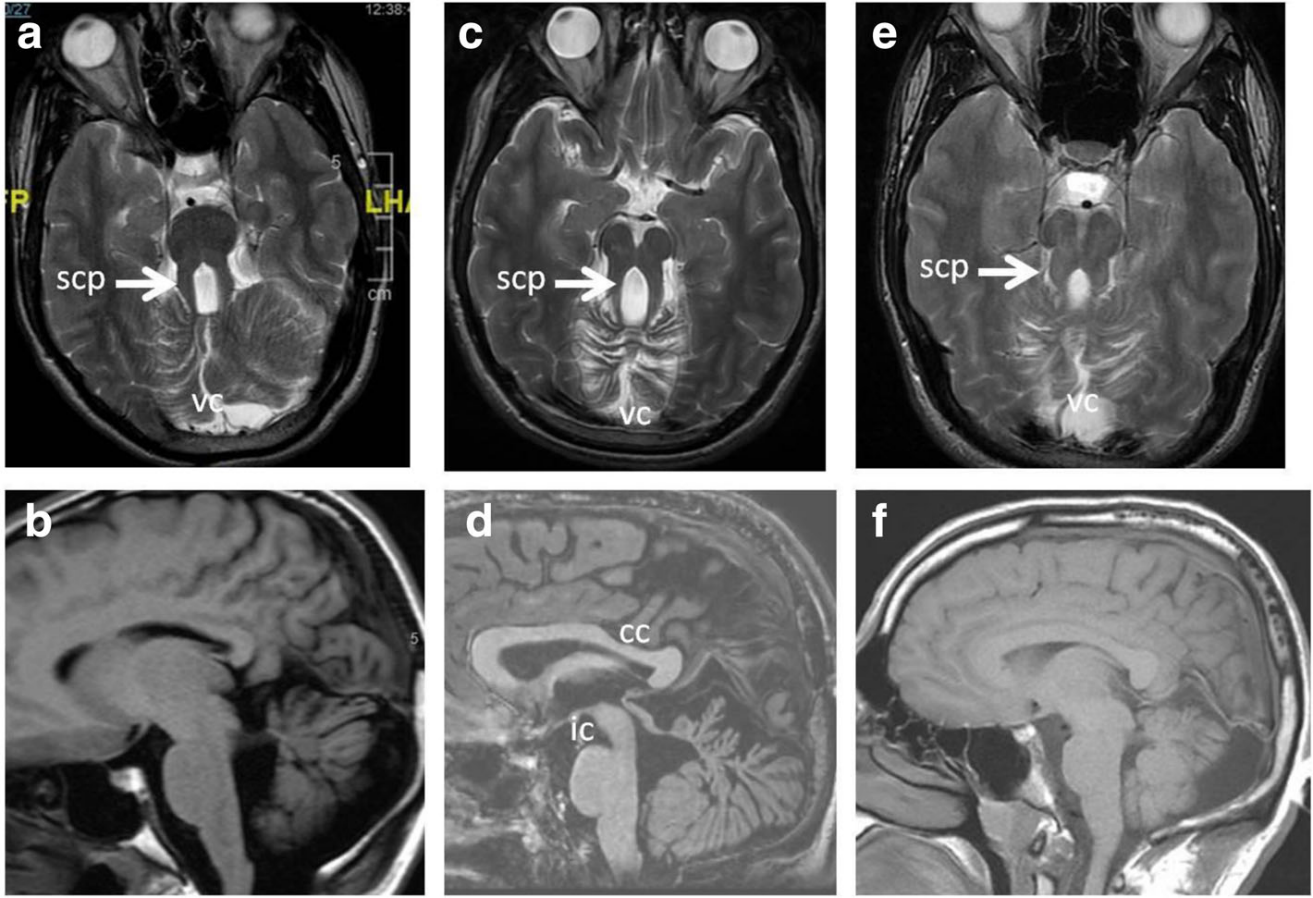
a-f MR images of the three patients with TMEM67 mutations/Joubert syndrome 6 phenotype. a an axial T2, and (b), a sagittal T1 weighted images in case 1, show the elongated superior cerebellar peduncles (scp, white arrow) that exhibit a classic molar tooth configuration, vemian and cerebellar hemispheric dysplasia, and a characteristic midline vemian cleft (vc). c an axial T2, and (d) a sagittal T1 weighted images in case 2, show the elongated superior cerebellar peduncles (scp, white arrow), vemian dysplasia, a characteristic midline vemian cleft (vc), deep interpeduncular cistern (ic), and an unusually contoured corpus callosum (cc). e an axial T2, and (f) a sagittal T1 weighted images in case 3, show the elongated superior cerebellar peduncles (scp, white arrow), vemian and cerebellar hemispheric dysplasia, and the characteristic midline vemian cleft (vc)
